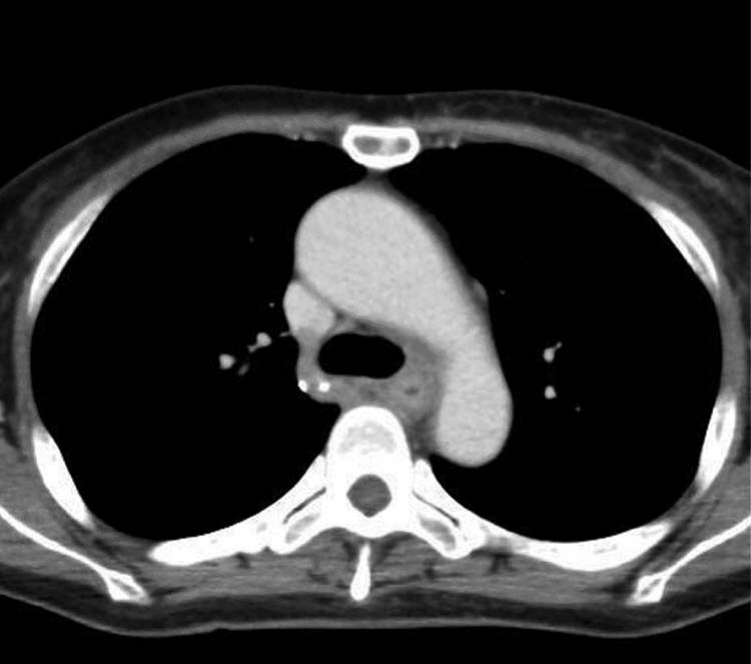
Coronal plane of the CT findings; CT demonstrates dilatation of the upper thoracic esophagus.
